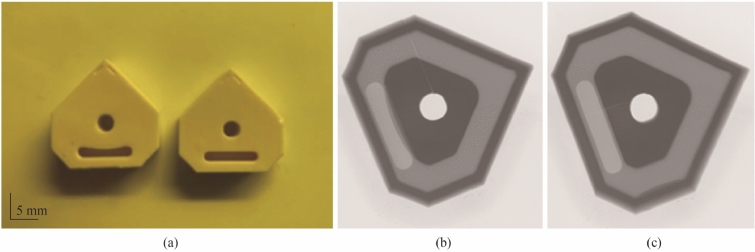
a Comparison of green bodies with the deformation displayed, b, c X-ray images of sintered inserts highlighting the geometrical deviation in b
